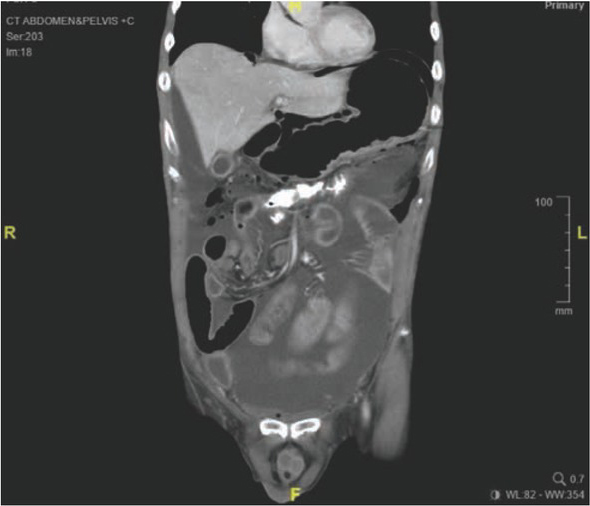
(Case 1) Two days after the distal loopogram was done, patient presented with generalised peritonitis and his CT abdomen showed gross ascites and pneumoperitoneum.
